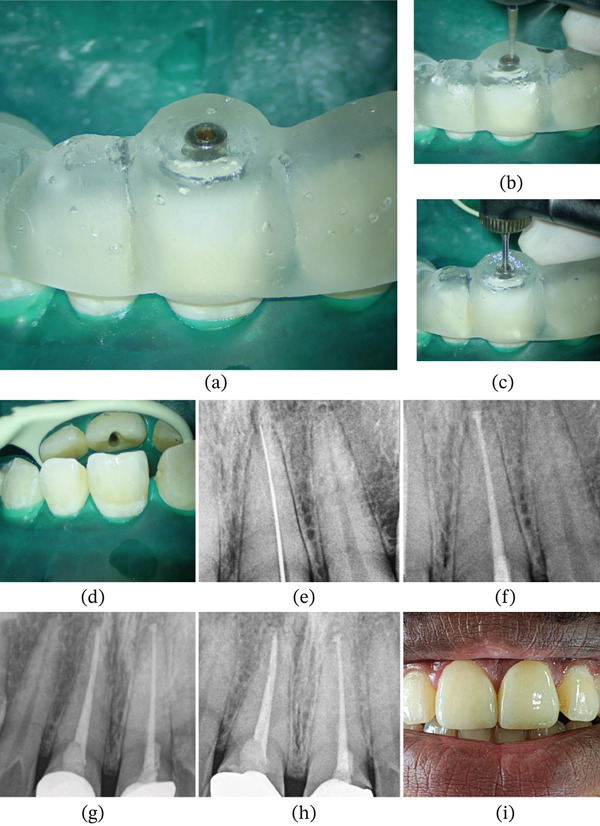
(a) Checking stability of the endoguide, (b) removal of enamel using high speed handpiece, (c) use of EndoGuide bur along the planned path, (d) conservative access achieved, (e) working length determined, (f) immediate postobturation radiograph, (g) 6‐month follow‐up radiograph, and (h–i) 12‐month follow‐up radiograph and clinical image.
